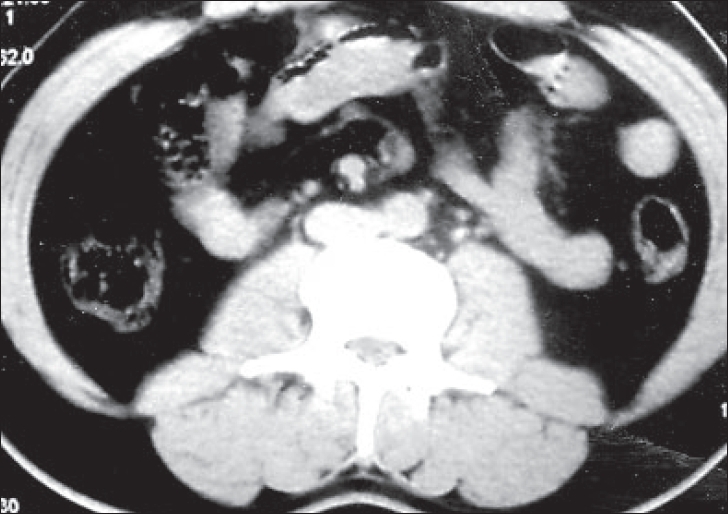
Non-contrast computed tomography showing right ureteric calculus
